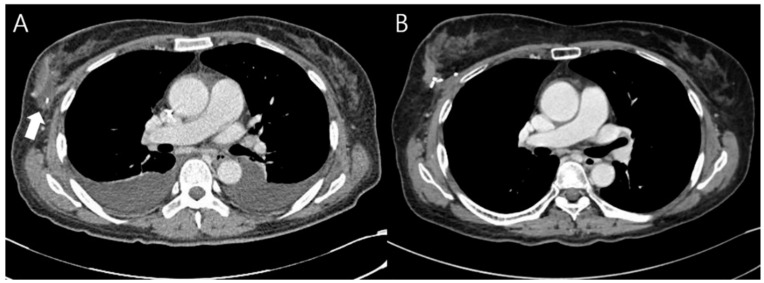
Chest CT image 3 weeks and 13 months after surgery. (A) A 54-year-old patient underwent breast reconstruction using the one ADM with the RBT after breast-conserving surgery. At 3 weeks after surgery, she complained of dyspnea during chemotherapy and a chest CT was taken. Seroma was noted on the chest CT (white arrow), and 5cc aspiration was performed, and was not observed when follow-up aspiration was performed 1 week later; (B) Seroma was no longer observed on the chest CT taken at 13 months after surgery.
